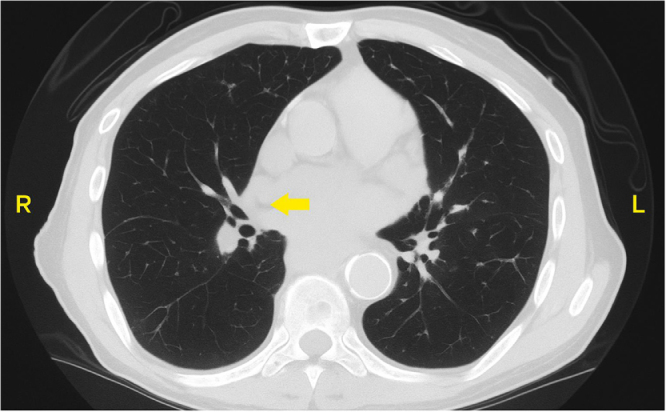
Chest CT with an irregular 7 × 8 mm pulmonary nodule along the right minor fissure.
